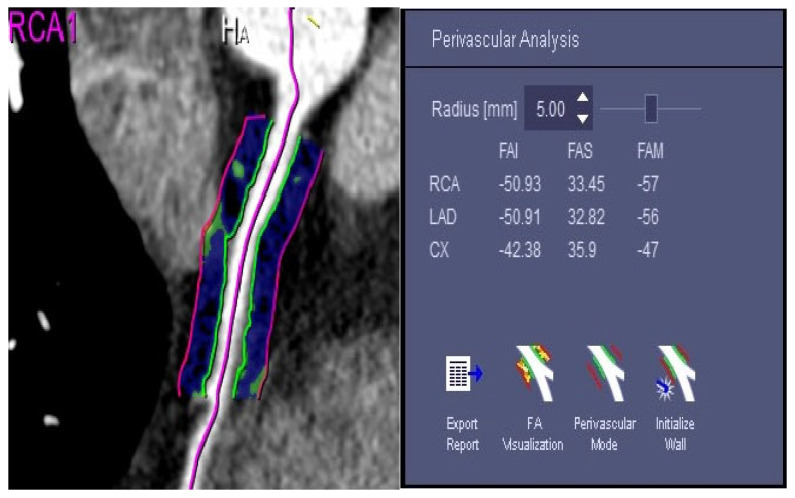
Measurement of FAI values: the target vascular segment was manually determined, and artificial intelligence software (CT Coronary Plaque Analysis, software version 5.0.2, Siemens-healthineers GmbH; Forchheim, Germany) was used to automatically outline the vascular wall (green line), and to automatically calculate the corresponding segment FAI value.
